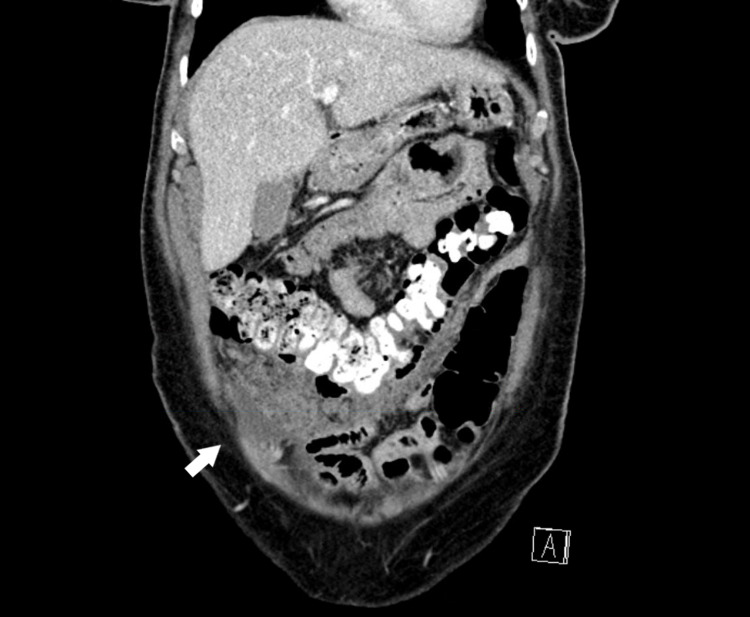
Contrast-enhanced computed tomography of the abdomen and pelvis Coronal section demonstrating omental caking concentrated in the right lower quadrant (white arrow).
